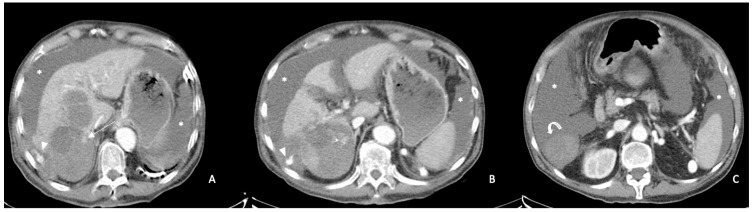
Arterial phase enhanced CT scan. HCC of the VII segment with necrotic intra-tumoral areas (arrows) and concomitant peri-hepatic and peri-splenic effusions (asterisks). Active contrast extravasation is visible near the tumor surface inside the hematoma (arrowheads (A,B)). The sentinel clot sign is also visible ((C), curved arrow).
